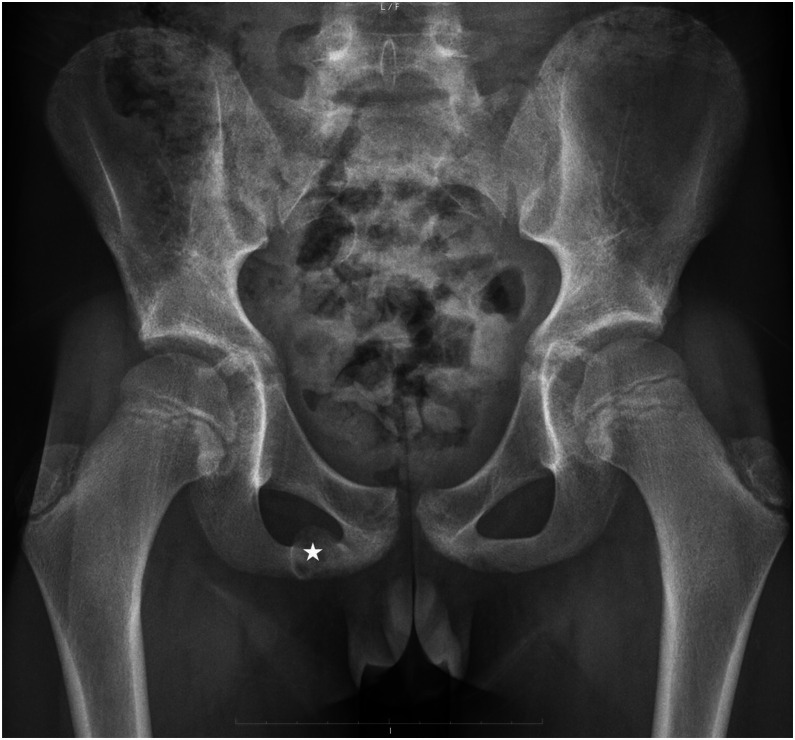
Pelvic radiograph showing a heterogeneous, expansile intraosseous lesion of the right ischiopubic ramus (asterisk).
